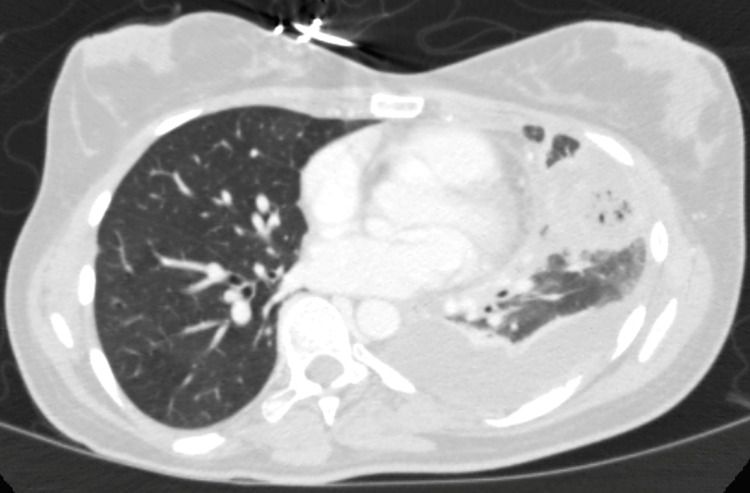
CTA chest (lung window) demonstrating left lower lobe necrotizing pneumonia with moderate-sized loculated left pleural effusion and abscess CTA: computed tomography angiography
